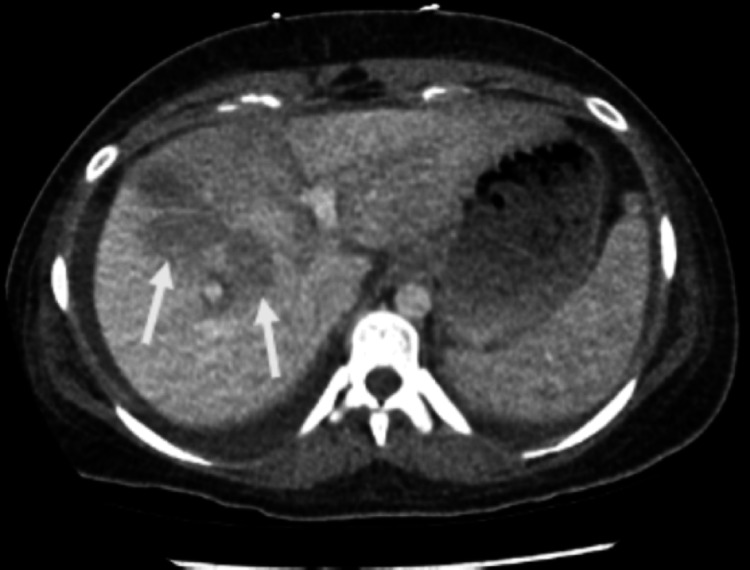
Axial view of a triple-phase CT abdomen with evidence of patchy necrosis indicated by arrows.
